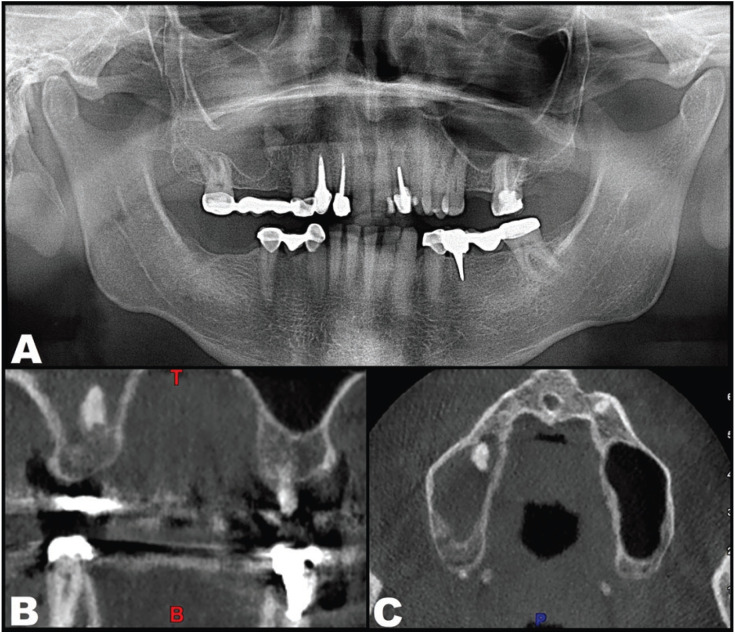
Initial radiographic findings. A. Panoramic X-ray view. B. Cone-beam computed tomography coronal view at the level of the root fragment displaced into the right maxillary sinus. C. Cone-beam computed tomography axial view at the level of the root fragment displaced into the right maxillary sinus.
